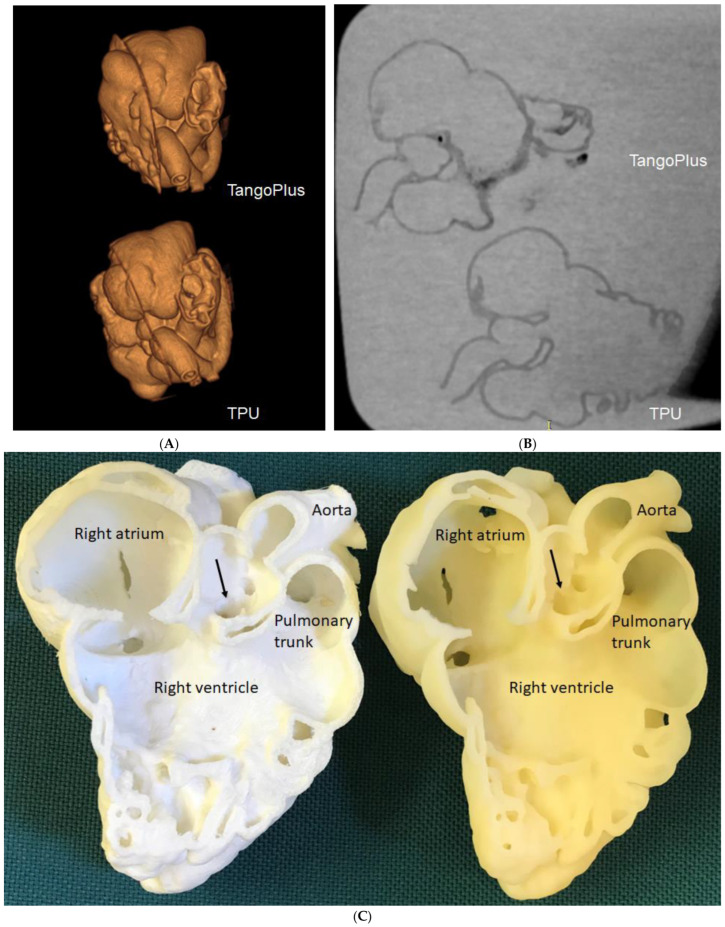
3D-printed congenital heart disease models with use of different materials for comparison of model accuracy. (A): 3D CT volume rendering of the 3D-printed models showing similar anatomical details. (B): 2D axial CT views of the 3D-printed models. (C): Inside view of cardiac chambers and aortic structures on both models. The white model was printed with TPU, while the yellow model was printed with TangoPlus. Arrows refer to subaortic ventricular septal defect. Reprinted with permission under the open access from Sun [51].
